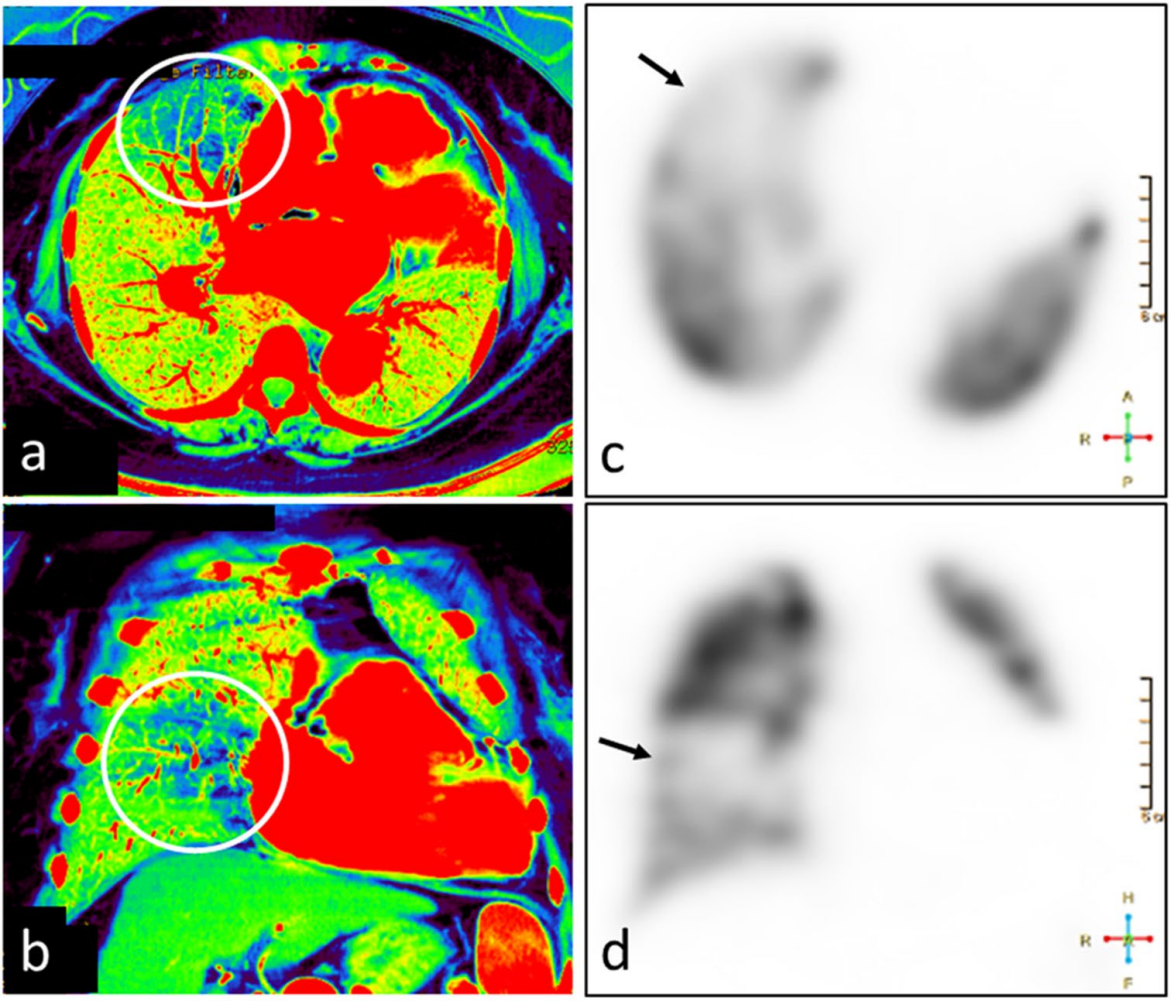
A 67-year-old woman with pulmonary-tumor thrombotic microangiopathy. The tumor embolism was too small for its detection on the contrast-enhanced CT scan. Catheterization demonstrated tumor embolism. Iodine maps (a, b) show areas with decreased blood flow in the right lung (circle), a finding consistent with a defect on lung perfusion scintigraphy (c, d) (arrows)
